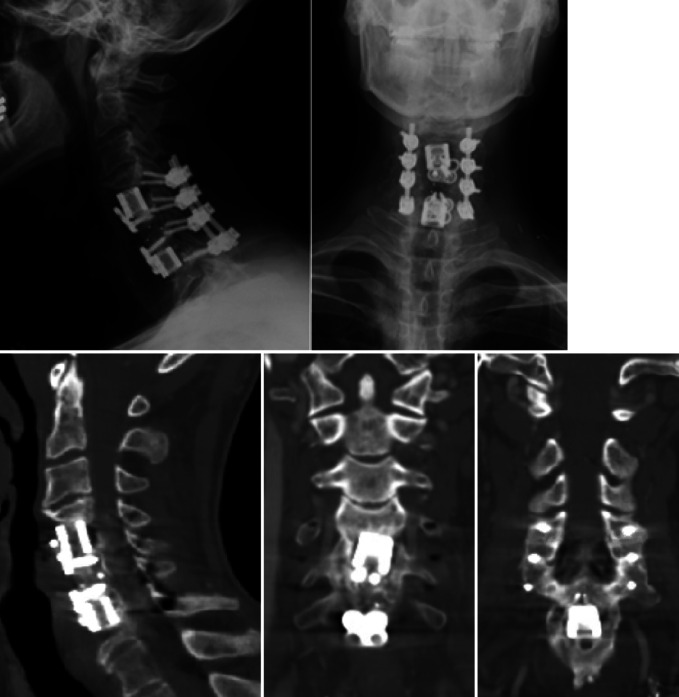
Cervical spine radiograph and CT performed 1 year postoperatively demonstrating C4-7 fusion with stable constructs and no signs of loosening or hardware failure.
